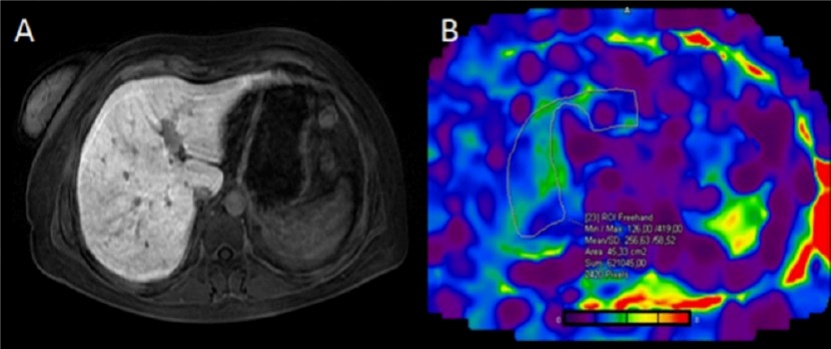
(A) MR image of the abdomen
